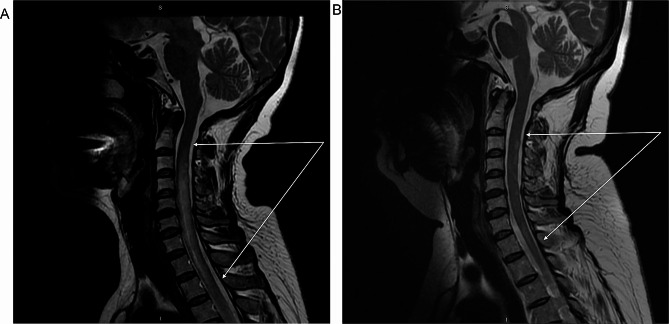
Magnetic resonance imaging (MRI) of the spinal cord. (A) MRI was performed when the patient was admitted to the hospital. It showed abnormal signals in the spinal cord at the C2 to T4 vertebral segments (arrows). (B) MRI reexamination after 4.5 months of treatments showing that the area of abnormal signals in the spinal cord has decreased (arrows)
